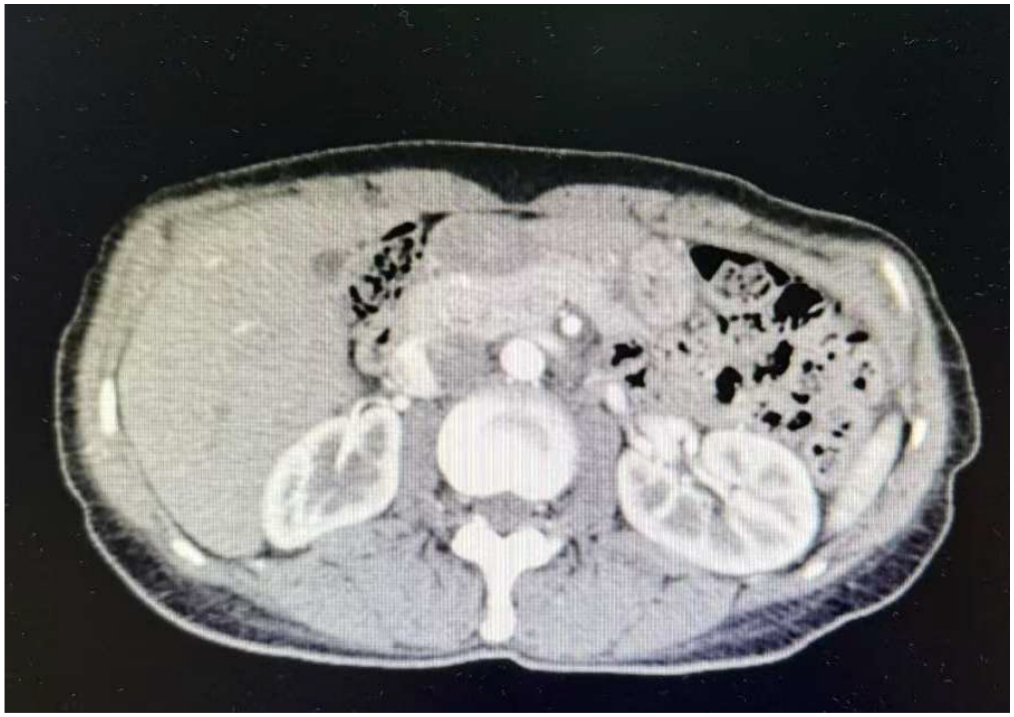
Contrast-enhanced CT of the adrenal glands. No obvious abnormalities were observed in the kidneys or adrenal glands. Multiple high-density lesions were detected in S5 of the liver, which were considered to be intrahepatic bile duct stones or calcifications.
